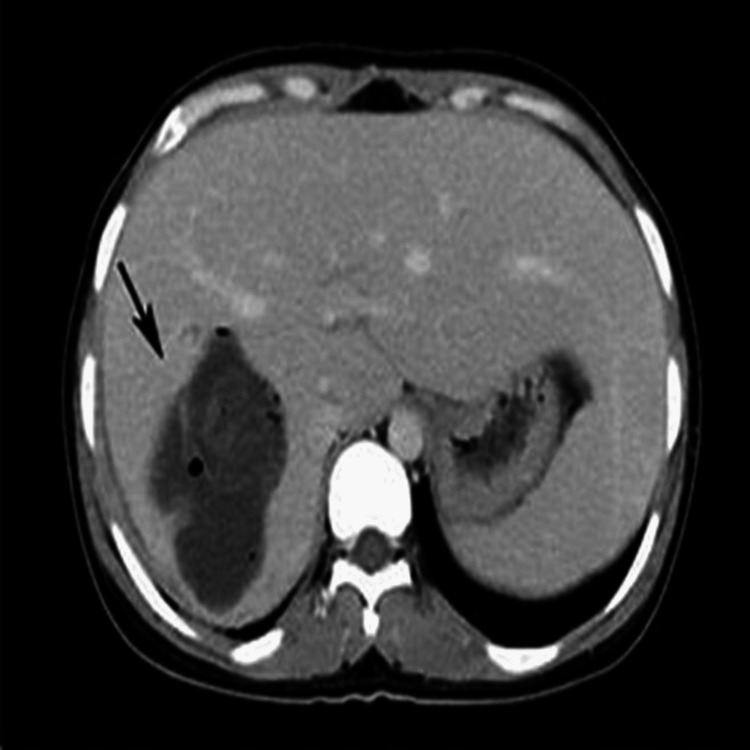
A CT scan (with contrast) of the abdomen An infected hydatid cyst of the liver in a 47-year-old woman. A contrast-enhanced CT scan shows a cystic lesion and collapsed membranes in the right lobe of the liver (black arrow).
